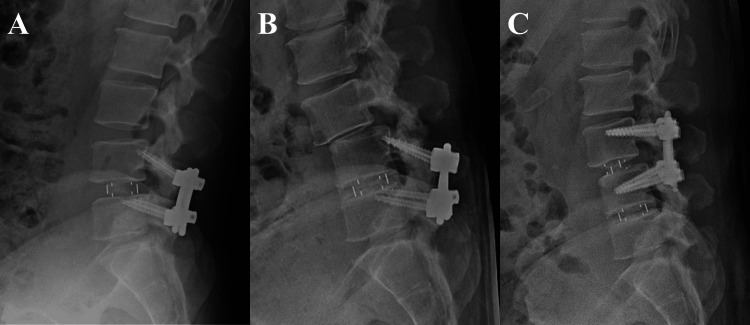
X-ray image of a representative case. A 49-year-old male (age at the time of re-OLIF). A: X-ray image after primary L4/5 OLIF; B: X-ray image taken five years postoperatively showing spondylolisthesis at L3 due to ASD; C: X-ray image after L3/4 re-OLIF with the removal of the L5 screw. OLIF: oblique lateral interbody fusion
